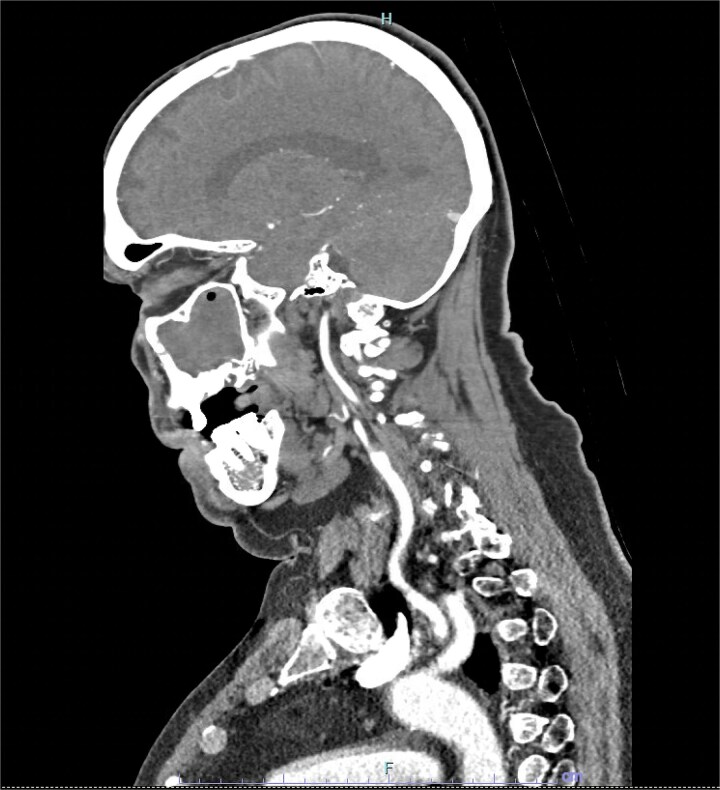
Computed-tomography-angiography (CTA) of the head and neck demonstrating no evidence of hemorrhage, infarct, or large-vessel occlusion.
